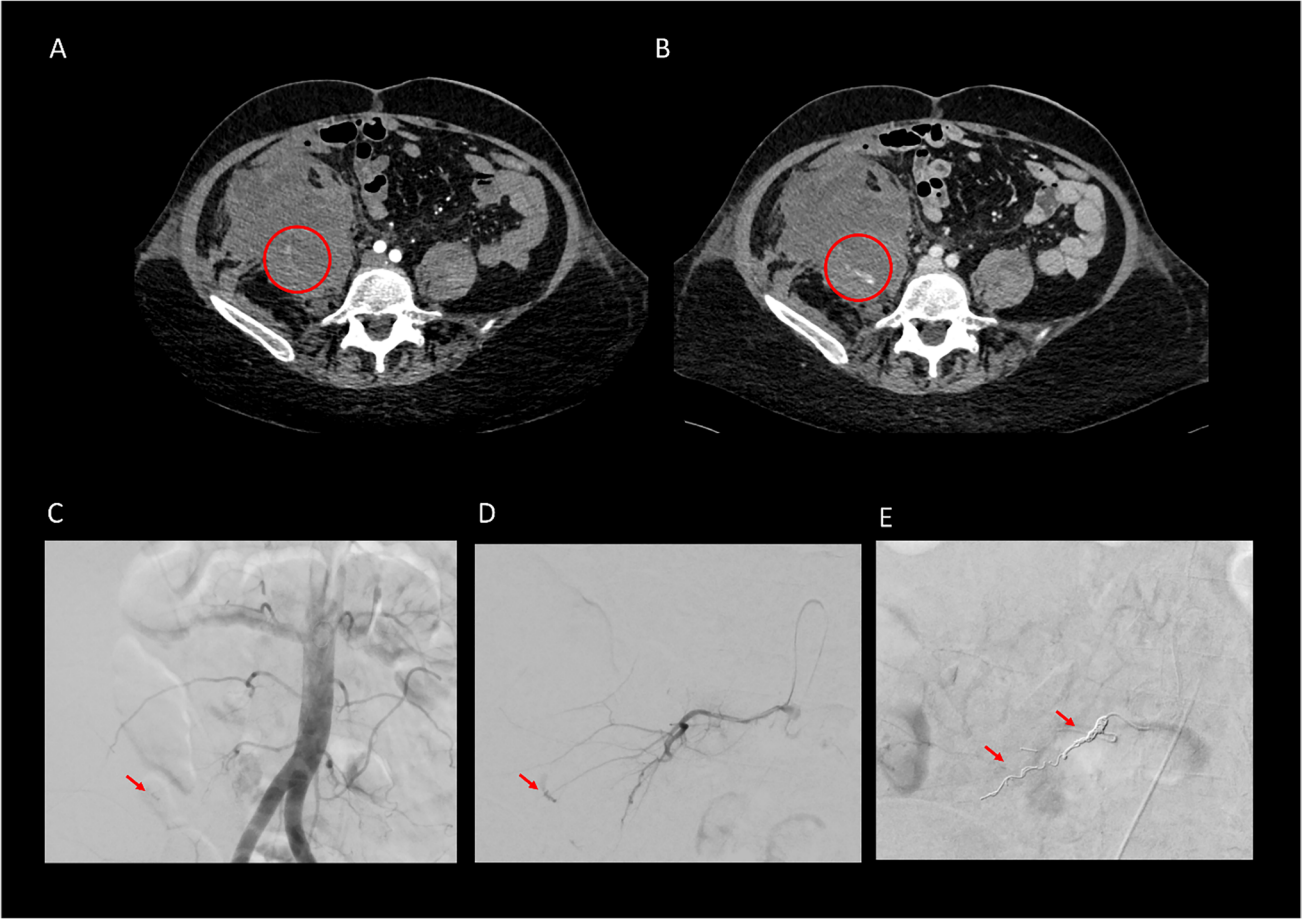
Image example of a patient (52y, f) presenting with hypotension (blood pressure 70/45 mmHg) and abdominal pain. Pre-interventional CT in transversal/coronal/ sagittal planes in the arterial (A) and venous phase (B) showed an active contrast extravasation in the right iliac muscle as well as a large retroperitoneal hematoma with signs of sedimentation. DSA (C) demonstrated an active bleed in iliolumbar branches of the right L4 artery (C, D), which had been suspected from the CT and which was consecutively embolized with a total of 22 coils (E)
